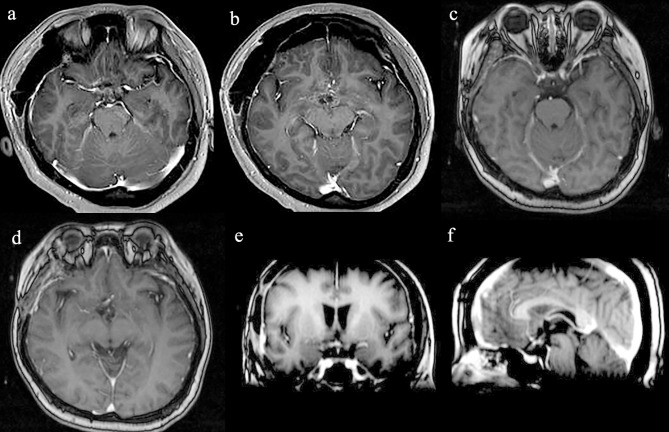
Radiological evaluation of CG postoperatively. Post-contrast MRI images of the residual tumor 1 month (a,b). The size of the residual tumor decreased following GKRS (c–f).
